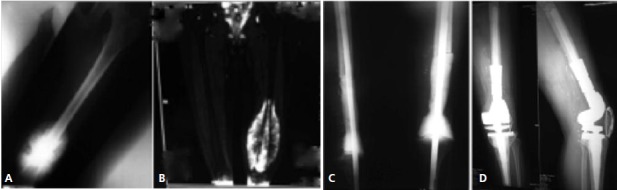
A. knee radiograph of a patient with osteosarcoma of the distal femur. B. MRI of the distal femur in the same patient C. 1st stage
